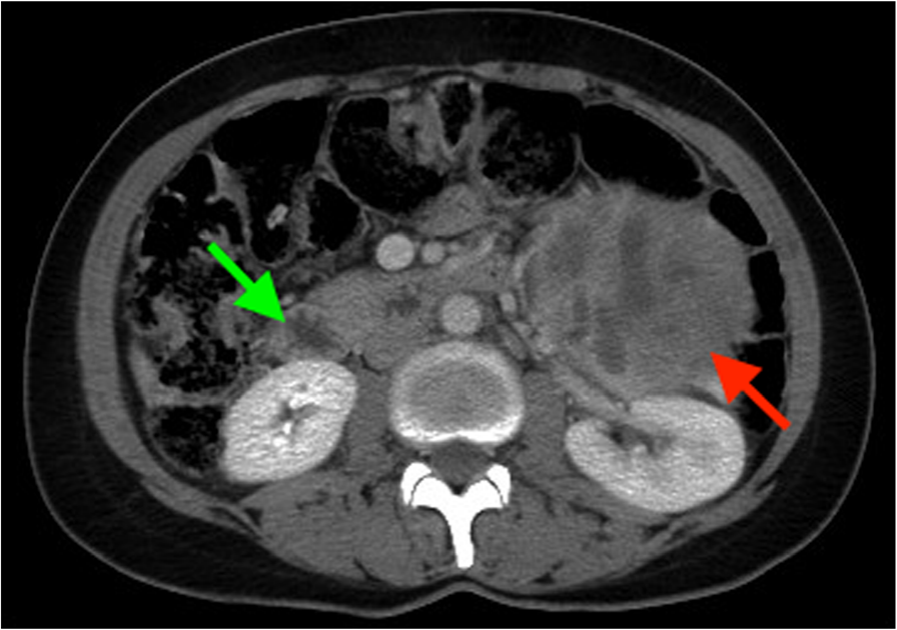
The abdominal computed tomography scan shows a left adrenal mass (*red arrow*) and a well-encapsulated nodule of the right adrenal gland (*green arrow*). The two lesions have central hypodense areas
